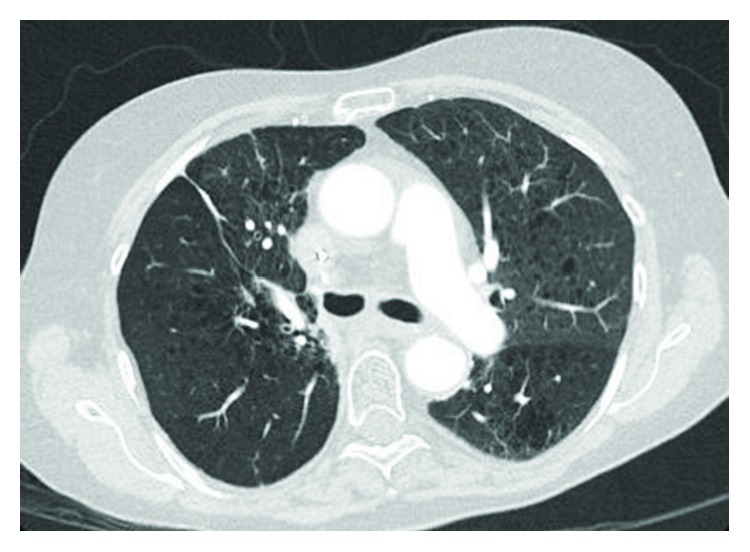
Axial CT chest with contrast approximately 3 months later in an outpatient follow-up, demonstrating a marked improvement of her infiltrate.
